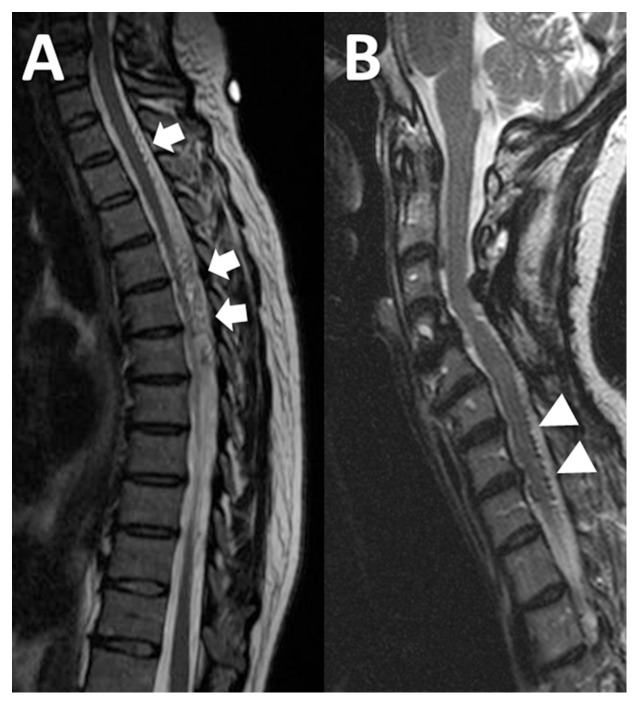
Abnormal flow voids without cord signal are seen in both patients with and without SDAVF. Sagittal T2-weighted images show prominent flow voids in (a) a patient with a positive spinal angiogram (arrows) and (b) a patient with negative angiography results (triangular arrowheads).
